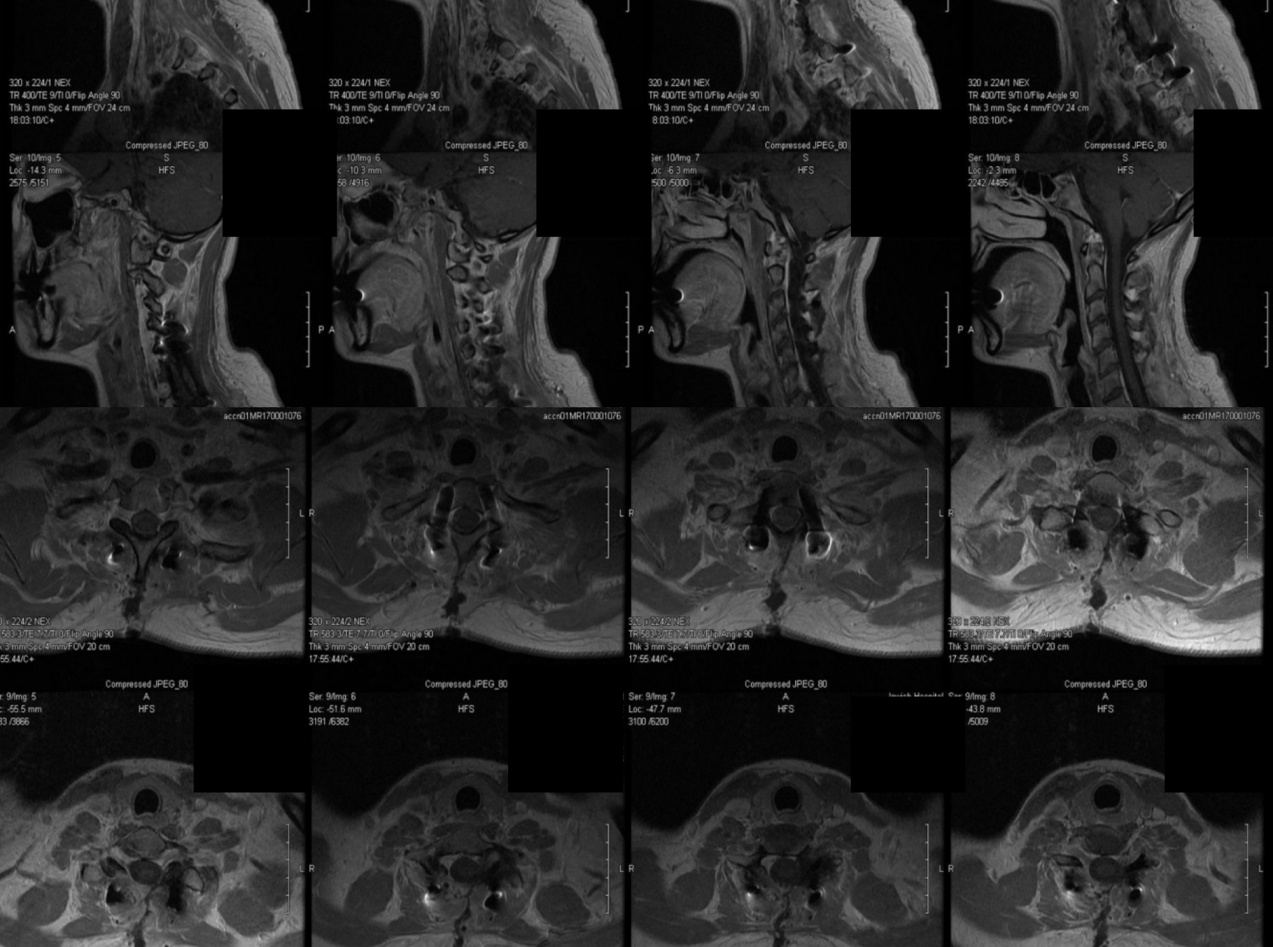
Postoperative MRI after the second surgery Postoperative contrasted MRI sagittal and axial after the second operation demonstrating gross total resection of the tumor MRI: magnetic resonance imaging
